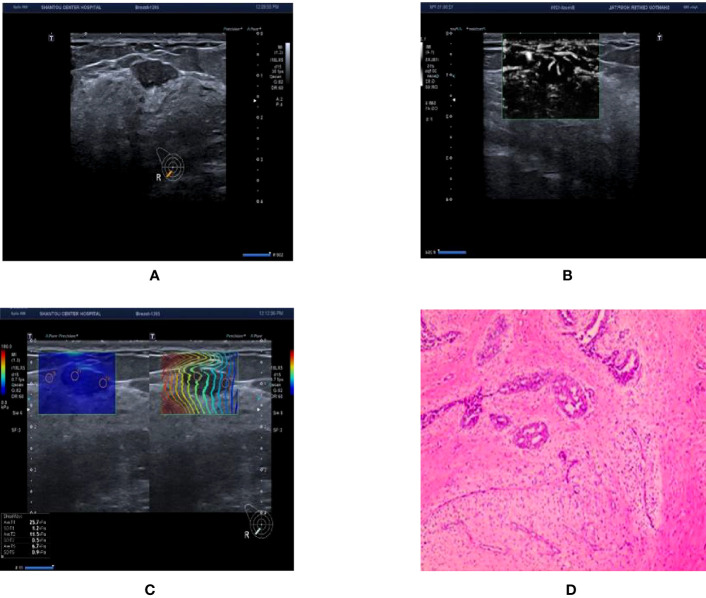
Ultrasound and pathological graphs. A 28-year-old female patient with a right breast nodule received ultrasound and pathological biopsy examinations. (A) A regular low-echo nodule was detected in the right outer and lower mammary quadrant, with parallel growth, lobed edges, even internal echo, and clear boundaries, BI-RADS 4a. (B) mSMI shows that microvessel morphology is dendritic subtype; BI-RADS grade was downgraded 1 level. (C) Shear wave elastic imaging: Emax is 25.7kPa < 77.25 kPa; BI-RADS classification was lowered or remained unaltered. (D) The nodule is a breast fibroadenoma based on pathological results.
